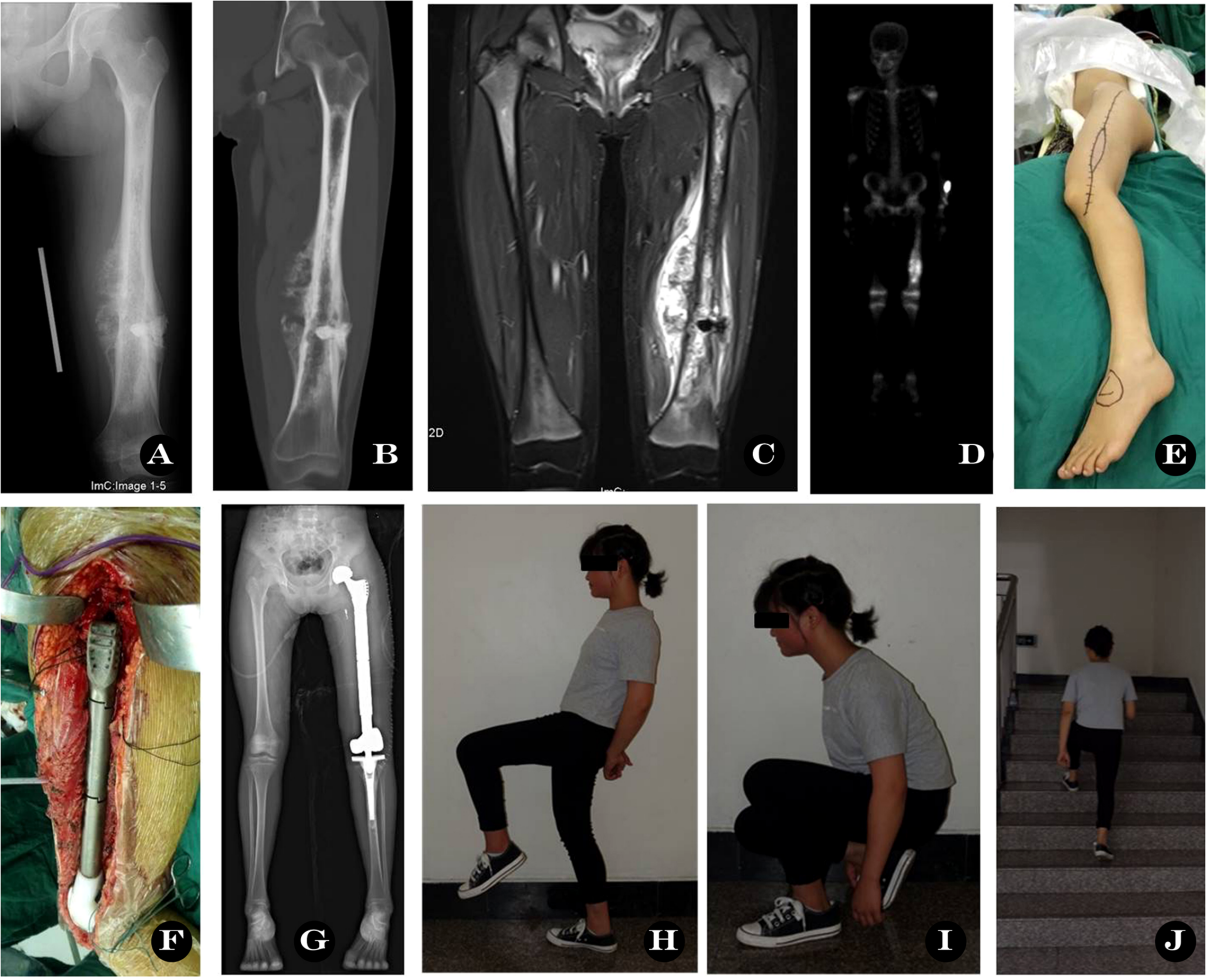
Total femoral prosthesis replacement. A 15-year old girl with left femur osteosarcoma. (A) X-ray before operation, (B–D) CT, MRI and SPECT image showing massive involvement, (E) Surgical incision, (F) Total femur prosthesis in operation, (G) X-ray post-operation, (H–J) Functional outcome at 33 months.
